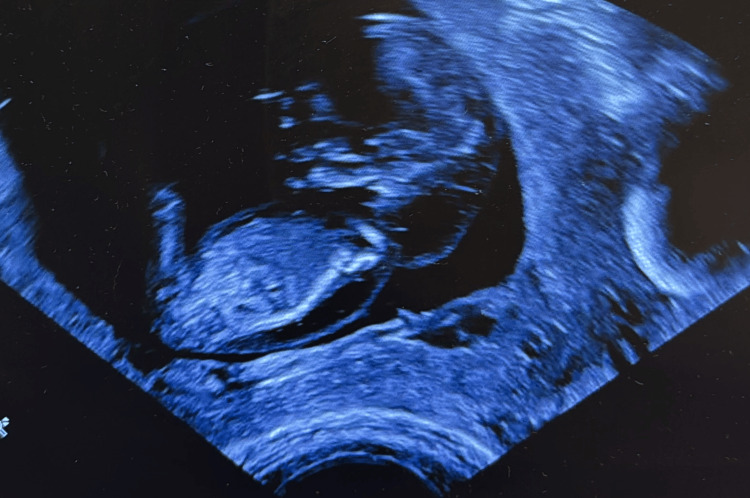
Nuchal translucency on prenatal ultrasound.
